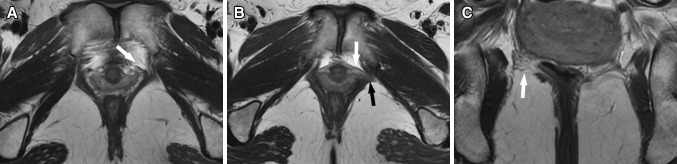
T2-weighted MR images of pubococcygeus and iliococcygeus tears. In a, an axial image from a 26-year-old primiparous woman shows partial (<50 %) left pubococcygeus avulsion near the pubic bone (*white arrow*); the right pubococcygeus muscle bulk is normal. In b, an axial image from a 31-year-old primiparous woman shows partial (<50 %) left pubococcygeus avulsion near the pubic bone (*black arrow*) with bilateral spilling of the vagina; the vaginal spilling on the left side is more severe (*white arrow*). In c, a coronal image from a 26-year-old primiparous woman shows partial right iliococcygeus tears near the obturator internus fascia (*white arrow*)
